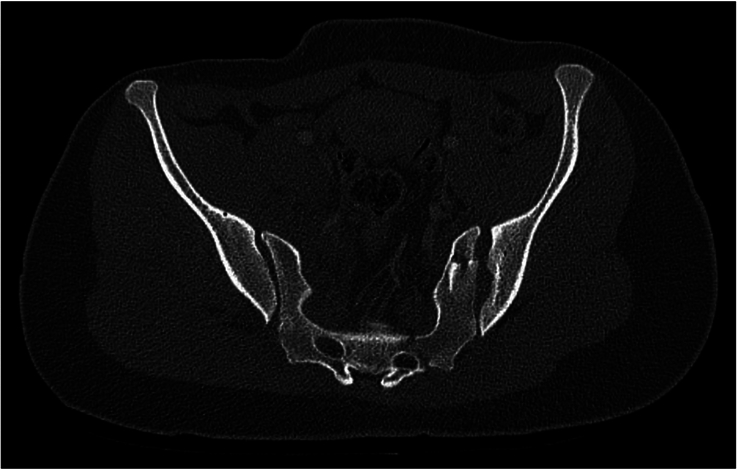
This CT scan demonstrates a pediatric LC3 (lateral compression type 3) fracture pattern in the left hemipelvis. The image shows a zone 2 sacral fracture, an iliac avulsion fracture, and significant widening of the sacroiliac (SI) joint. These features are indicative of a severe lateral compression injury, characteristic of an pediatric LC3 fracture pattern in a pediatric patient.
